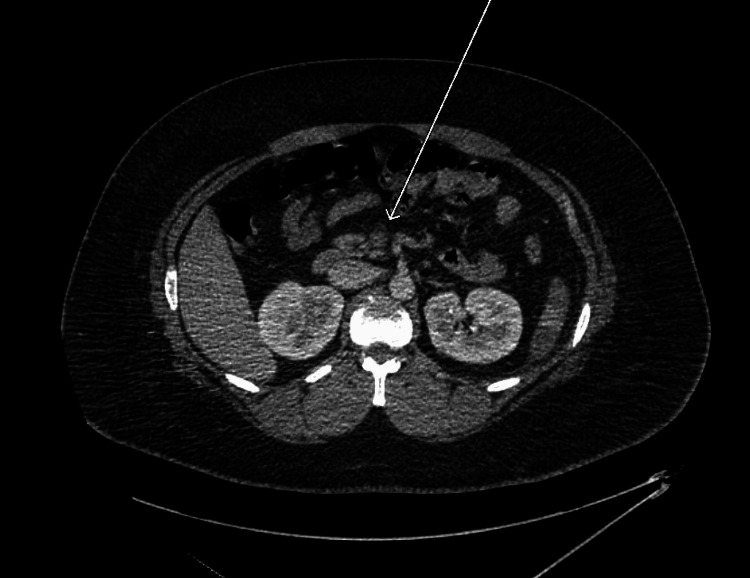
Axial CT image with intravenous contrast demonstrates a filling defect within the splenic vein (arrow), consistent with splenic vein thrombosis CT: computed tomography
